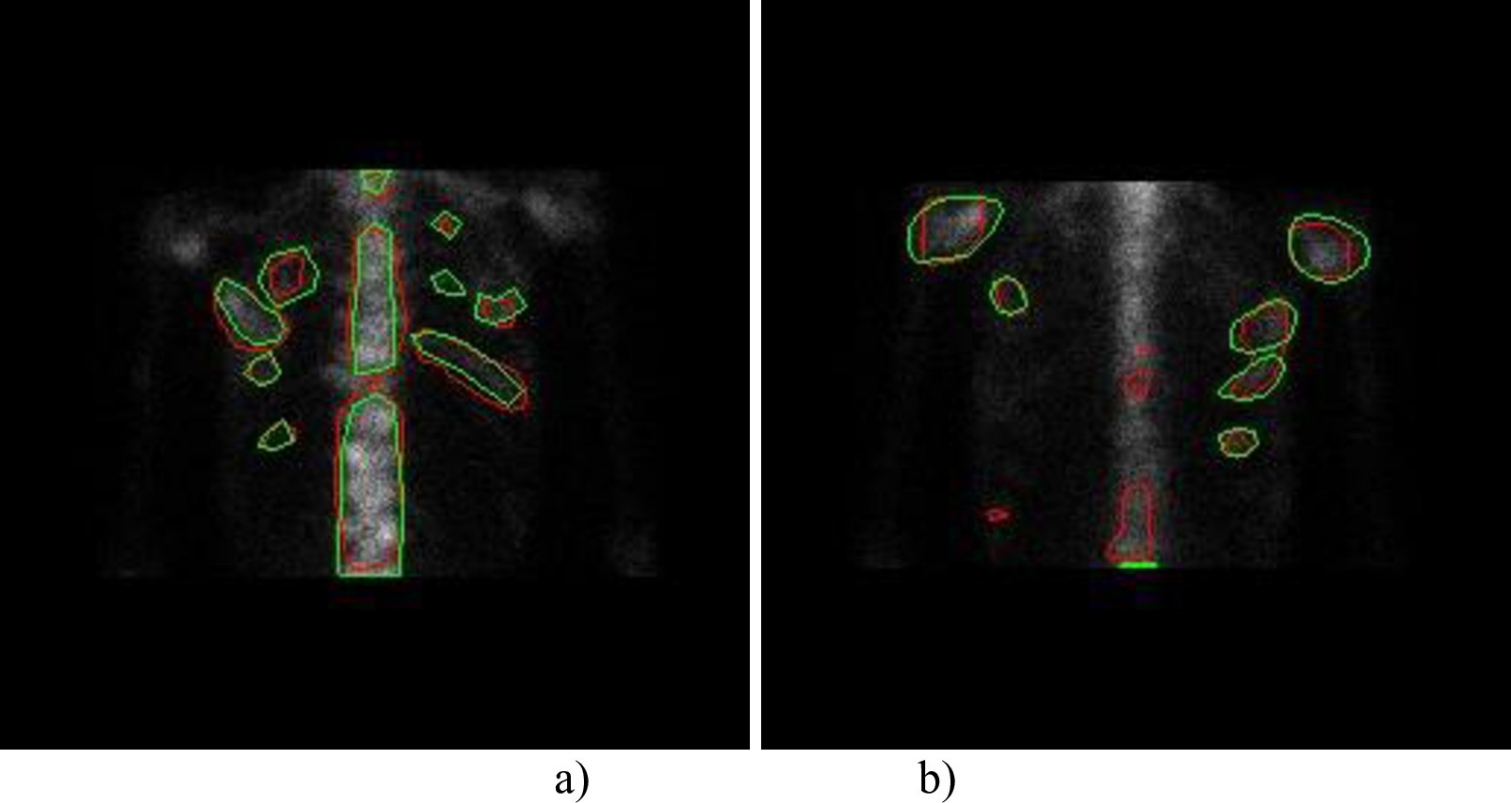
A visualization of hotspots segmented by U-Net-Res model for two thorax bone SPECT images (the model segmented results marked with green and the manually labeled ones marked with red). a) The best case; and b) The worst case.
